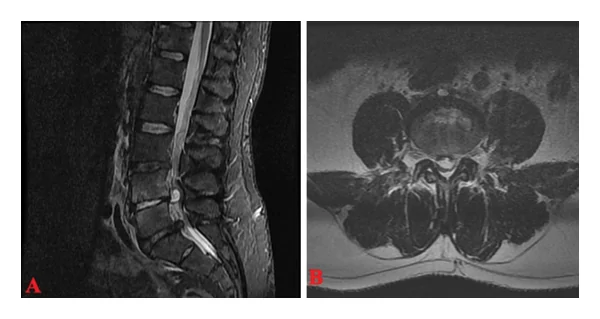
Preoperative magnetic resonance imaging (MRI) revealed a 1.6 × 1.1 cm lesion at the L4–L5 level with associated Type I modic changes (A). Additionally, MRI showed another view of the lesion along with a central disc herniation at L5–S1 causing moderate spinal stenosis (B).
